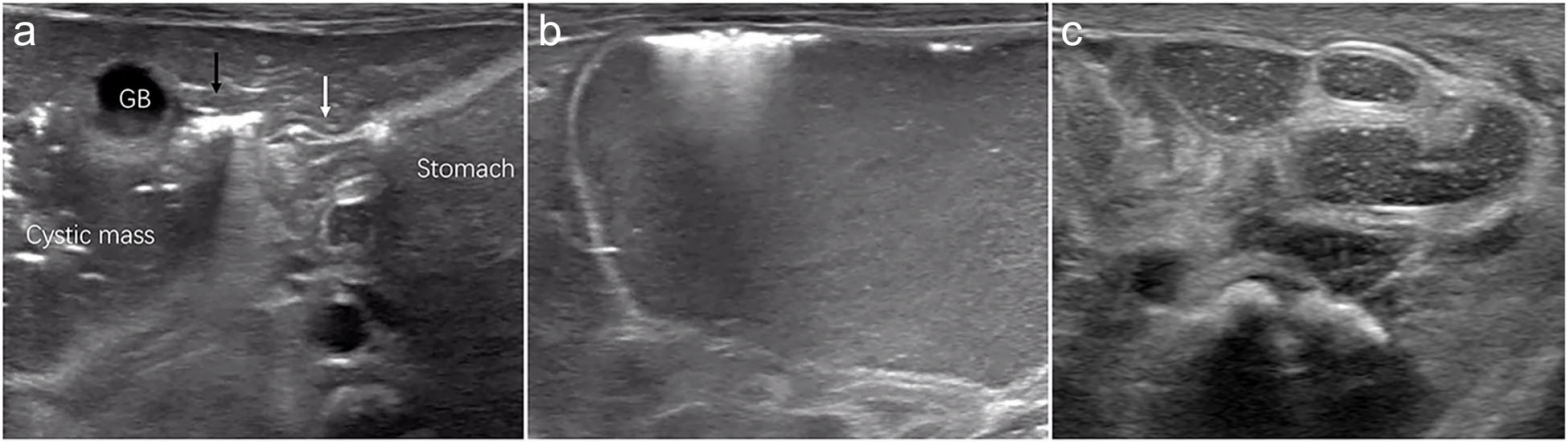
Ultrasound images of the child at 10 h after birth. a The cystic mass is connected to the stomach through the pylorus (*white arrow*) and upper duodenum (*black arrow*). The cystic mass and gas-like echoes are seen in the upper duodenum. b A giant cystic mass, approximately 7.3 × 5.1 × 3.9 cm in size, is located in the right abdominal cavity and has ground-glass echogenicity. Gas-like echoes also appear in the cystic mass. c The colonic bowel is compressed to the left side of the abdomen. No obvious abnormalities in the colon and rectum are seen
